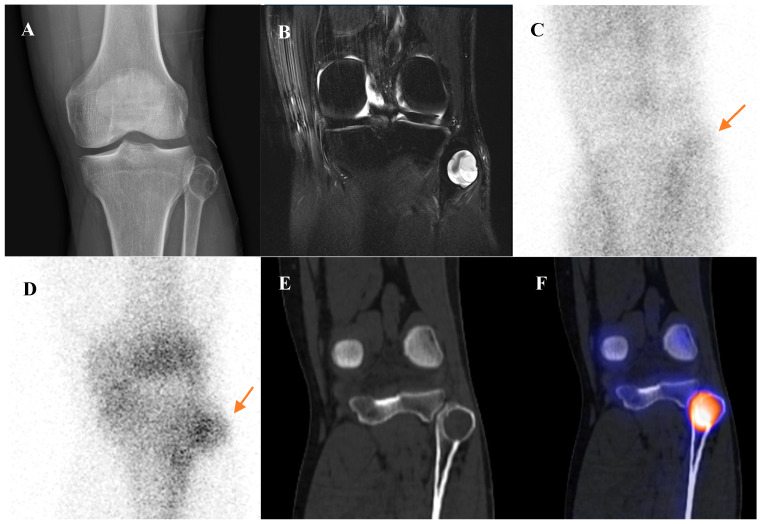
A 45-year-old male with an aneurysmal bone cyst (ABC) of the left proximal fibula. (A) Plain radiograph shows a sharply defined, expansile, lucent lesion of the proximal fibula. (B) T2-weighted fat-suppressed magnetic resonance imaging demonstrates a multiloculated cystic mass with preserved cortical continuity but bulging deformity. Fluid–fluid levels are present within the chambers, without solid enhancing components, and overlying soft-tissue swelling is noted. (C,D) Bone scintigraphy reveals mildly increased perfusion on the blood pool phase and focal uptake on the delayed phase (arrows). (E,F) Bone single-photon emission tomography/computed tomography demonstrates tracer uptake predominantly along the cystic lesion margin. The lesion was surgically excised and confirmed as ABC.
